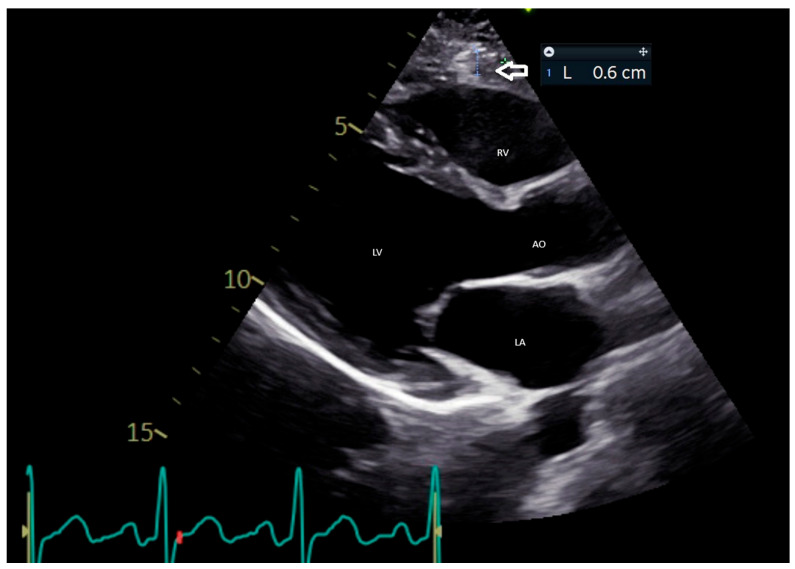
Echocardiographic parasternal long-axis view showing measurement of epicardial fat thickness. AO: aortic root; LA: left atrium; LV: left ventricle; RV: right ventricle.
